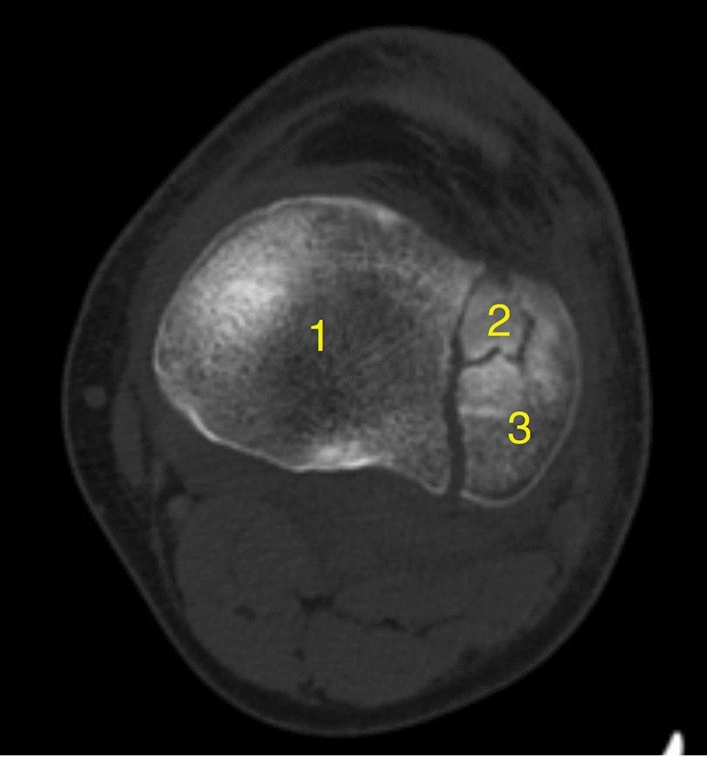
Axial CT of the tibial plateau demonstrating the measurement of the number of articular fragments
